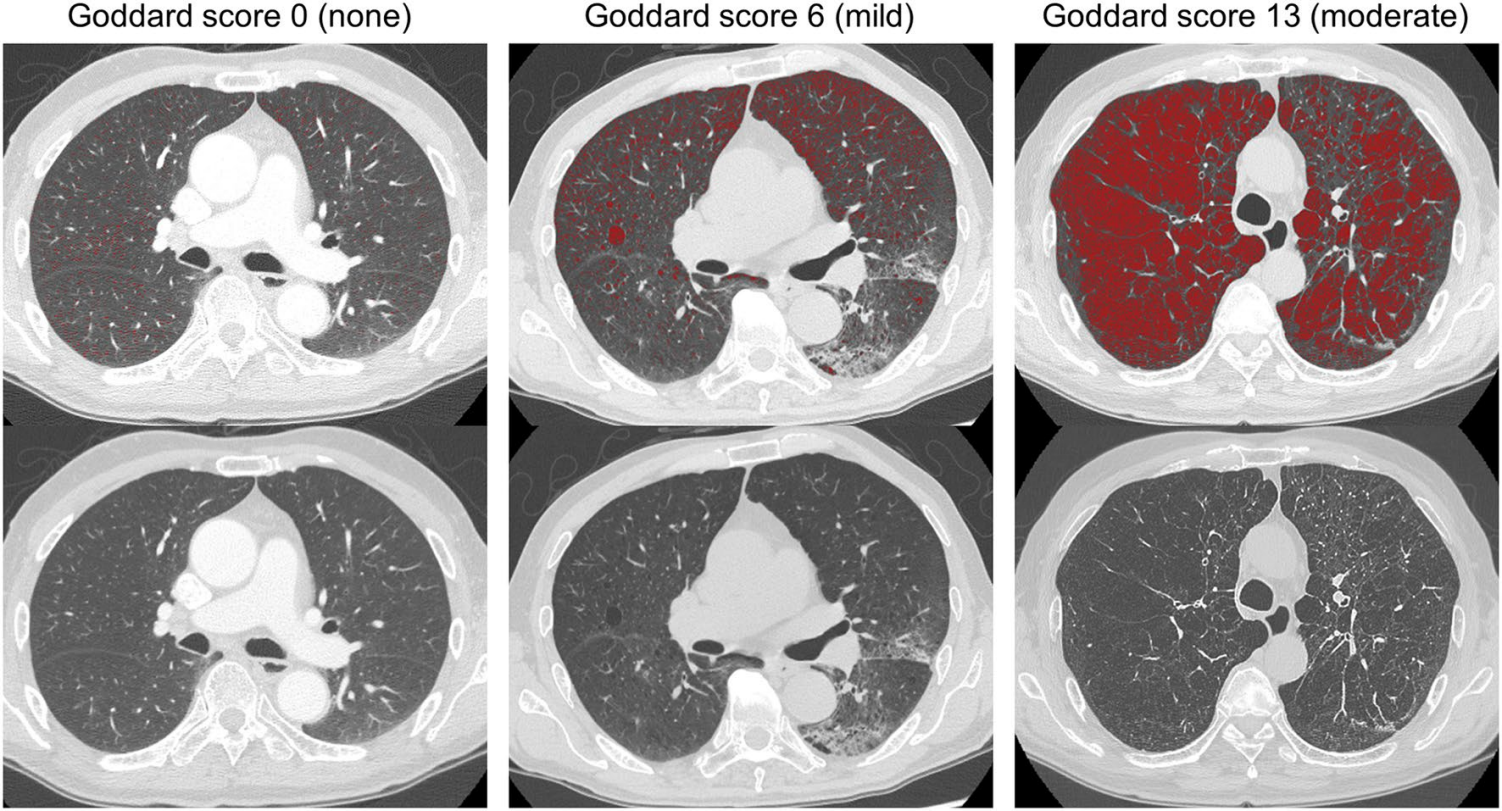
Representative computed tomography images showing the Goddard score using SYNAPSE VINCENT software (Fujifilm Medical, Tokyo, Japan).
